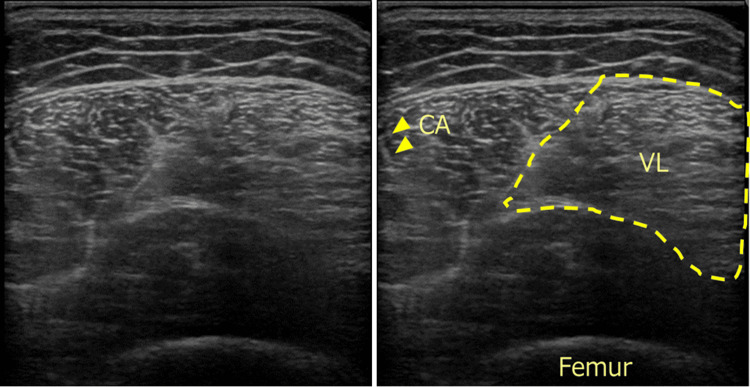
Representative ultrasound image of the rectus femoris. Representative ultrasound image of the rectus femoris showing a deviation of >2.5 cm (right). The guide is shown in the left panel. In cases with deviations of >2.5 cm (33%), the VL occupies half of the image. Abbreviations: CA, central aponeurosis; VL, vastus lateralis
